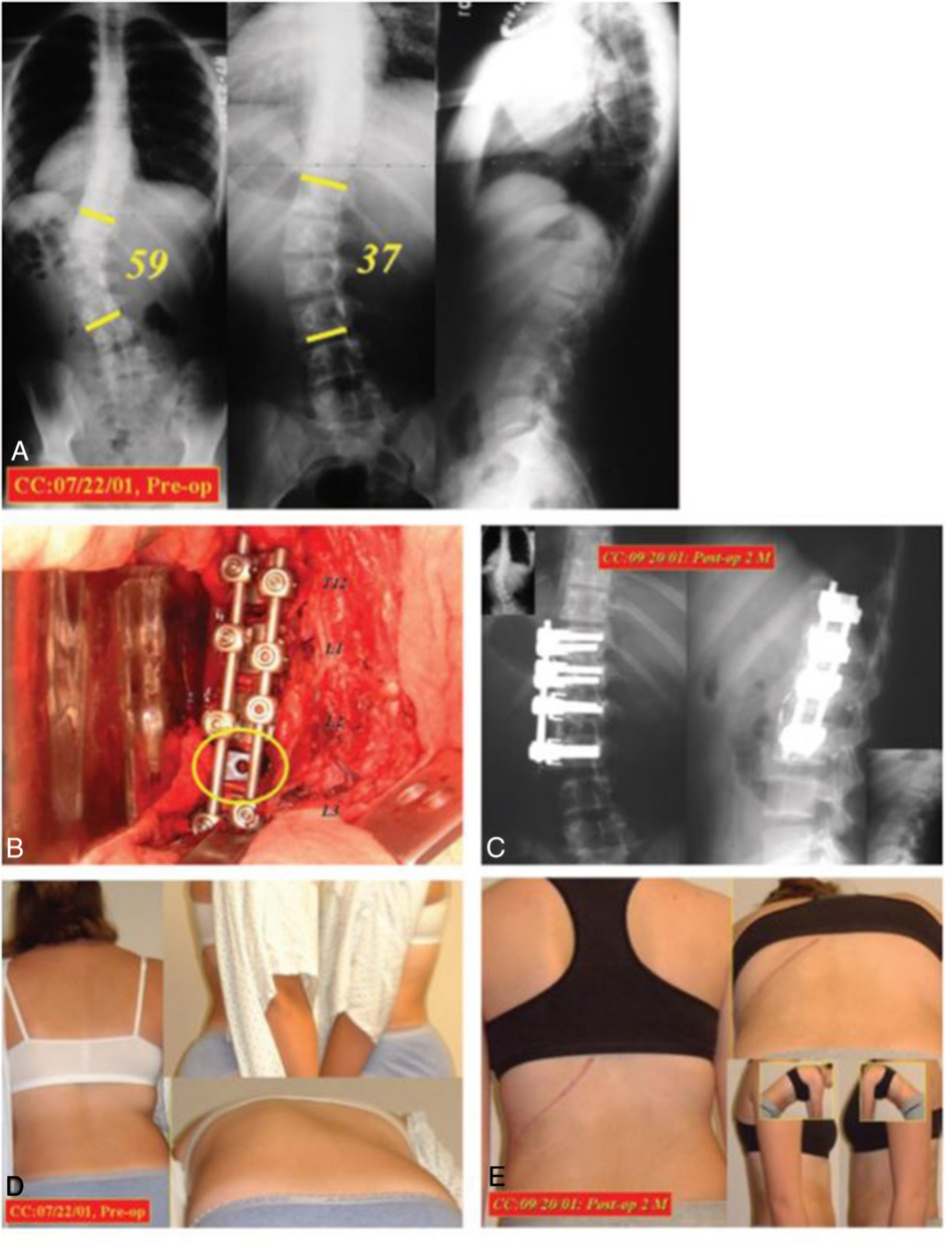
A, Posteroanterior and lateral preoperative standing radiographs of a 13-year- old girl with a 59° Lenke 5CN left thoracolumbar curve. The curve is reduced to 37° on the “stretch film.” There is thoracolumbar junctional kyphosis of 7° in the later instrumented segments T12 to L3. B, A carbon fiber cage is inserted into the L2–L3 disc space to improve the sagittal alignment. C, 2 months after anterior instrumentation, the entire thoracolumbar curve is reduced to 13° with the instrumented levels T12 to L3 improved to 4°. D, Presurgical trunk decompensation to the left with trunk rotation. E, View 2 months after surgical correction: the trunk is balanced over the pelvis; there is no more trunk rotation.
